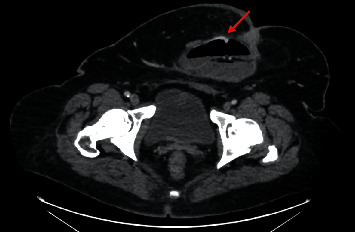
CT angiography showing a varix in the sac of the umbilical hernia.
